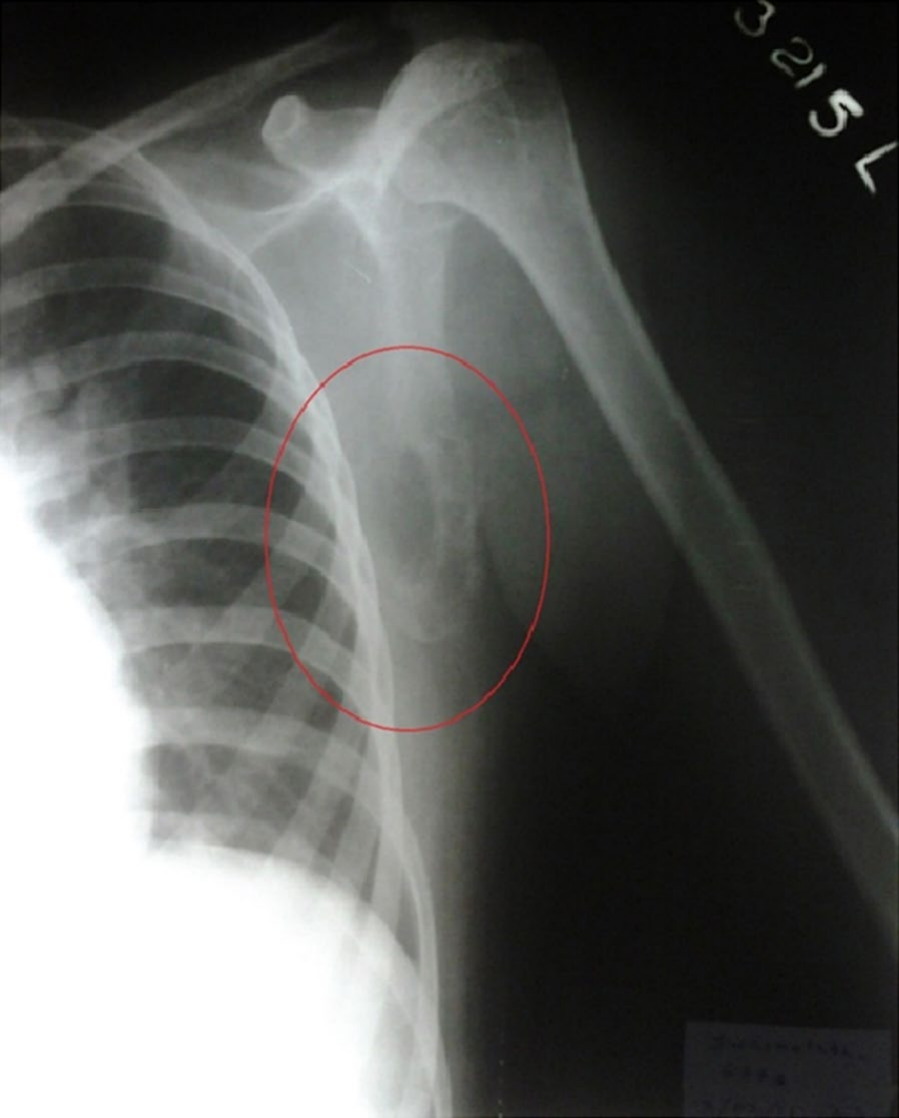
Radiograph of the *left* scapula. Radiograph of the *left* scapula showing a bony mass with multiple lytic lesions in the lateral and dorsal aspects.
